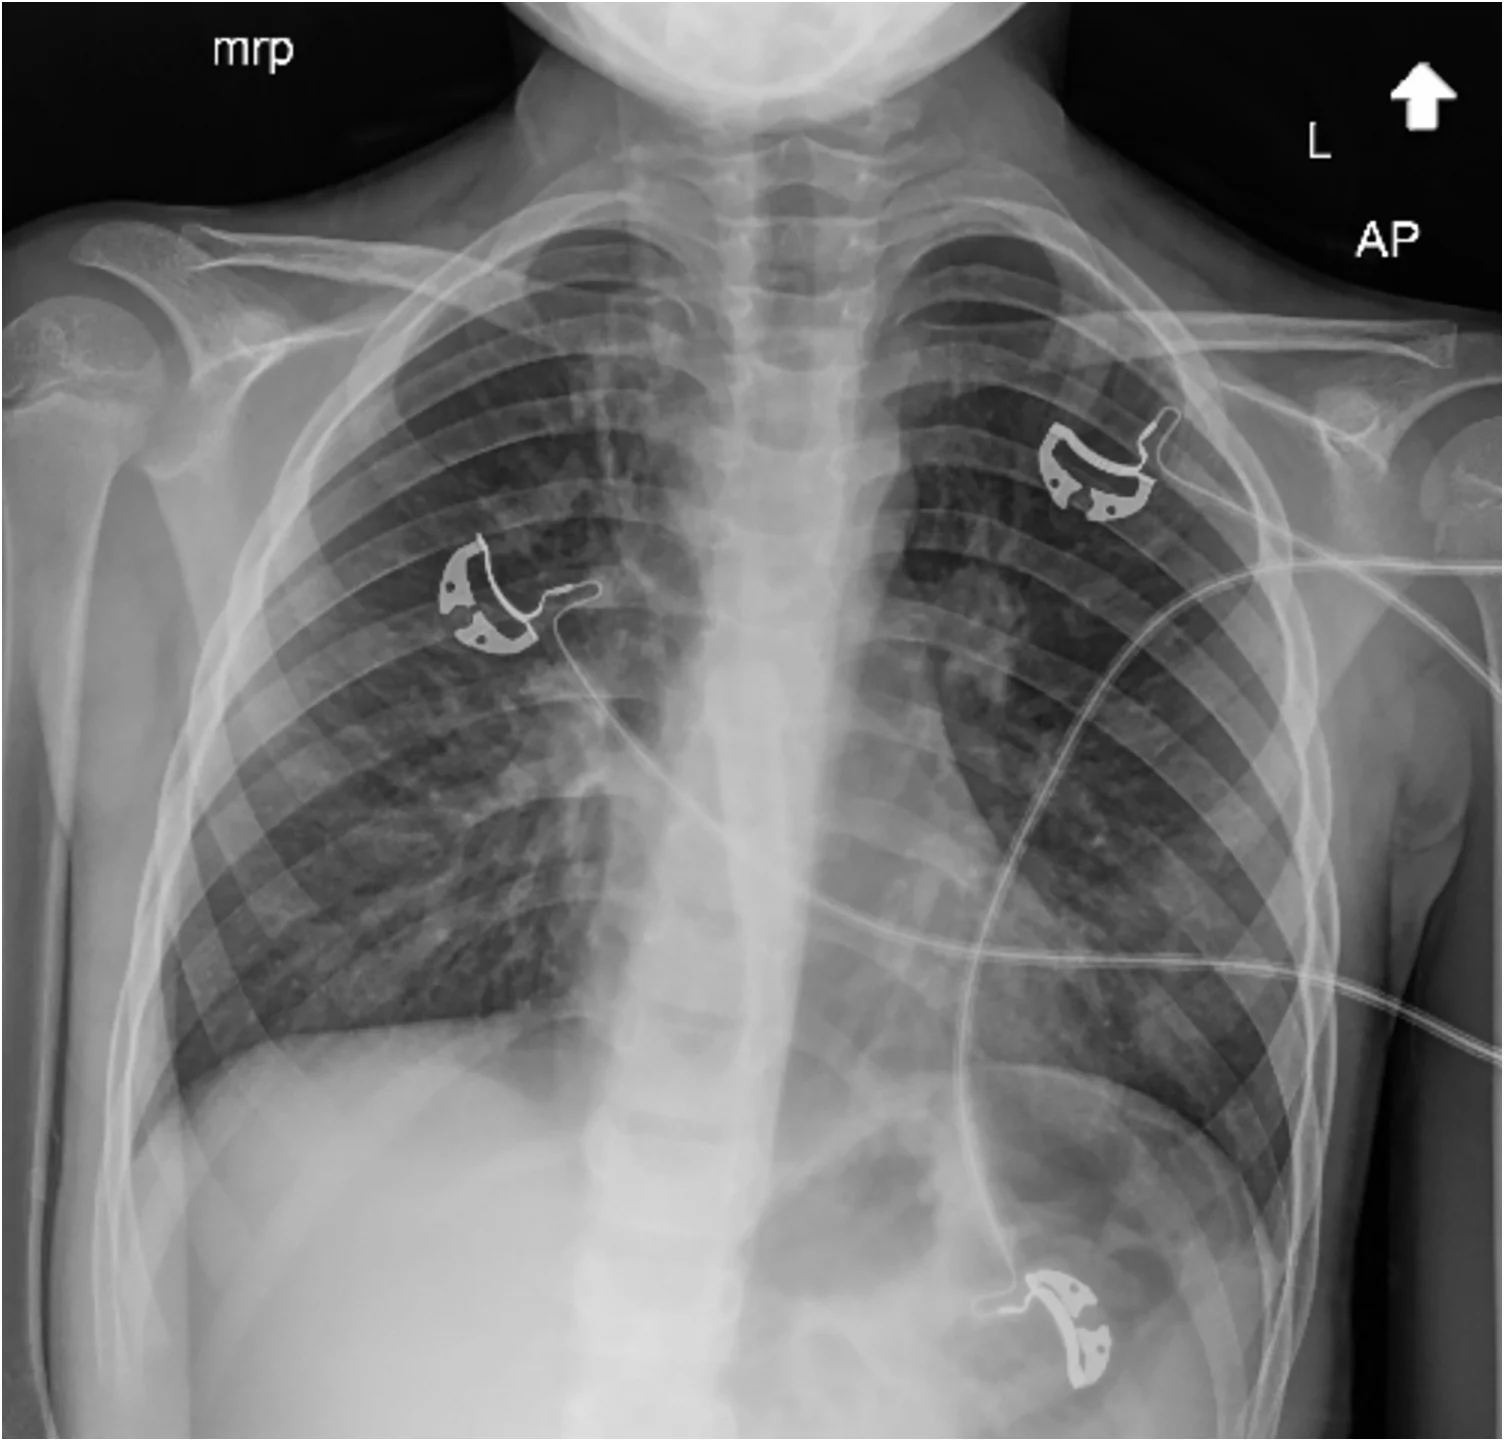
Chest radiograph - anteroposterior chest radiograph demonstrating a small patchy opacity in the medial right upper lobe. No pleural effusion or cardiomegaly is identified.
